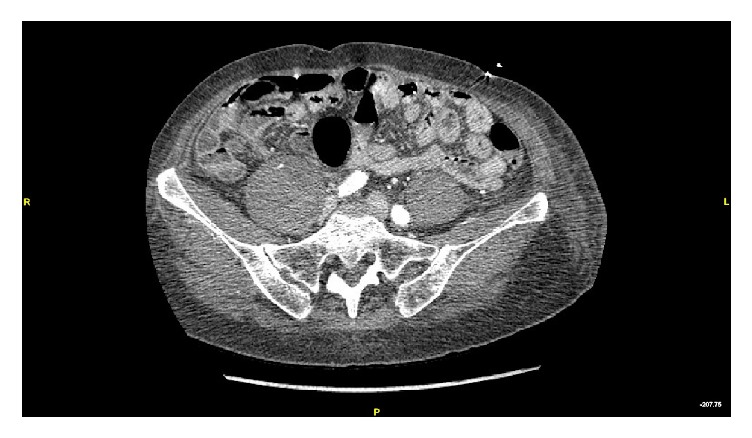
Computed tomography image with contrast in axial plane demonstrating right-sided iliopsoas hematomas as presented in case 2.
